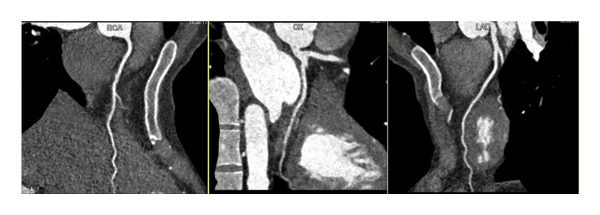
CCTA showed no significant coronary artery disease. (RCA: right coronary artery, LAD: left anterior descending artery, CX: circumflex artery, CCTA: coronary computed tomography angiography).
